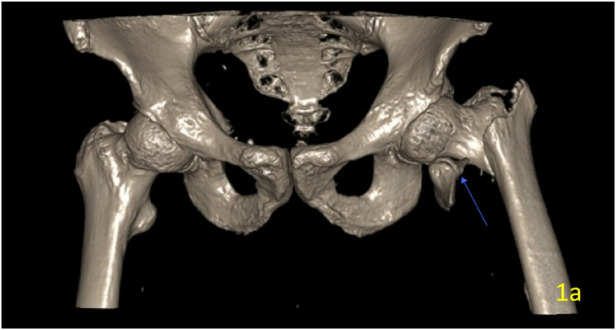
Preoperative 3D-CT reconstruction of the left hip. The image shows a markedly displaced intertrochanteric femoral fracture, classified as 31-A2.2 according to the Orthopaedic Trauma Association (AO/OTA 31-A2.2).
